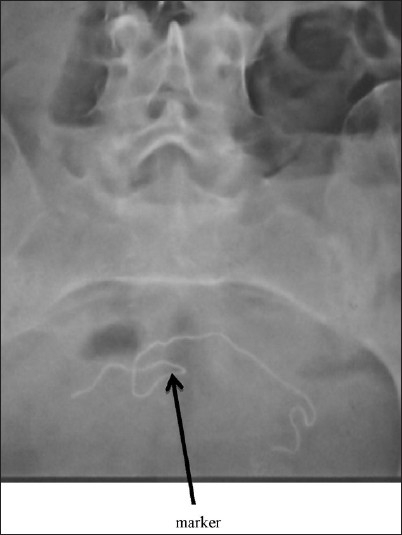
X-ray showing intestinal obstruction with marker of mop
